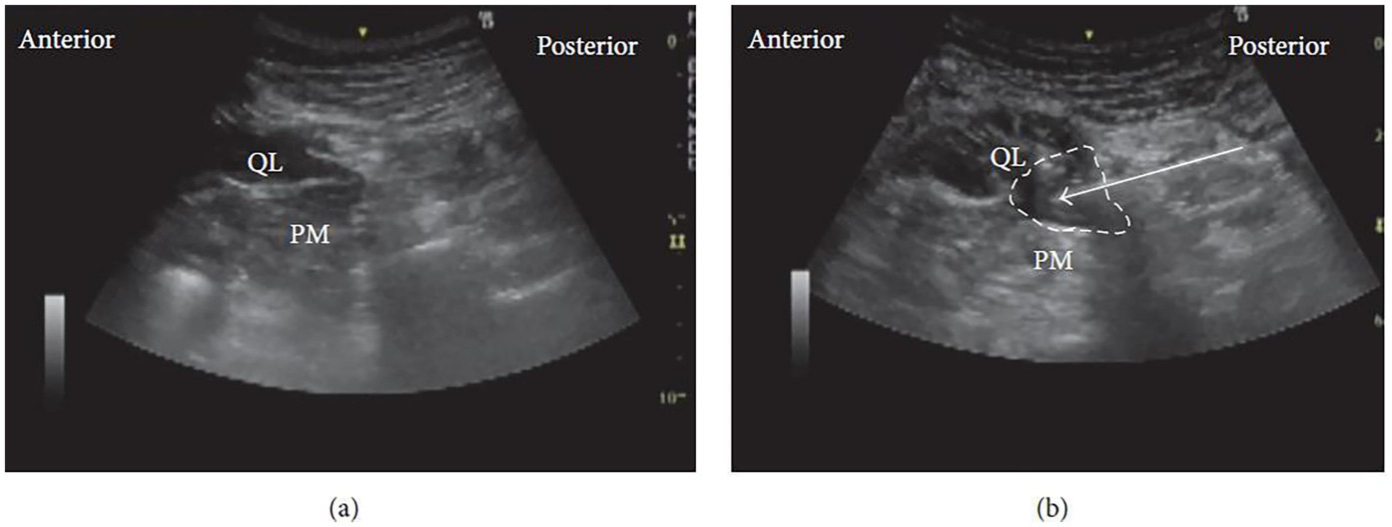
Ultrasound images of anterior QLB: (a) before injection and (b) after injection. QL, quadratus lumborum; PM, psoas muscle. White arrow: needle trajectory; white dotted line: spread of local anesthetic [Reproduced from Ueshima et al. (28). © John Wiley & Sons, Inc. Distributed under the Creative Commons Attribution License, CC BY 4.0].
